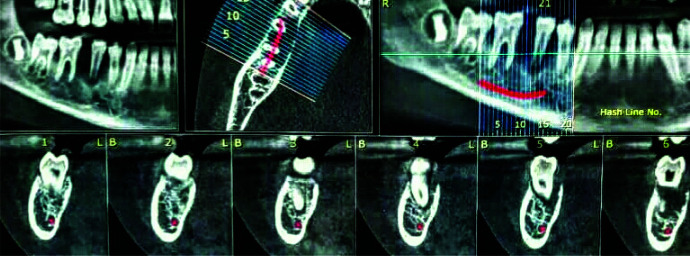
Cone beam computed tomography (CBCT) image; mandibular canal is intact
